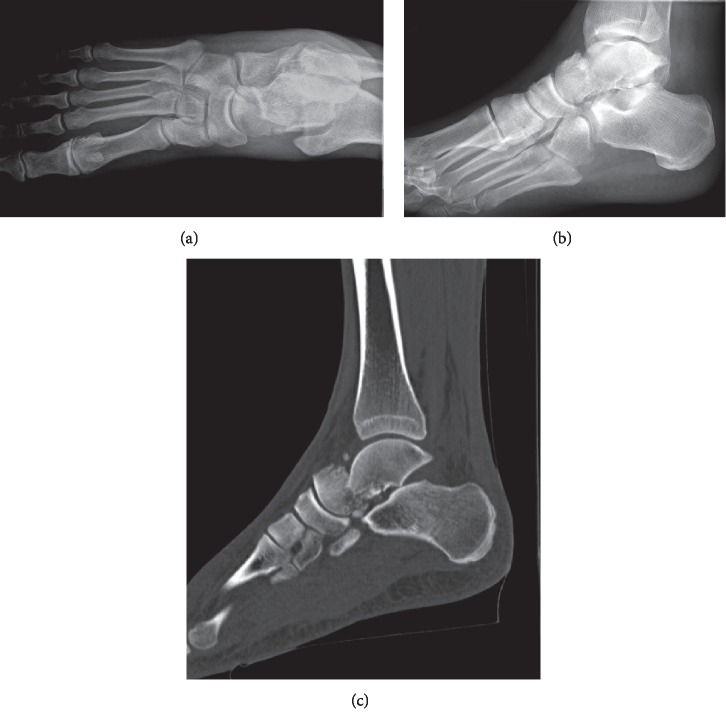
AP (a) and lateral (b) foot X-rays demonstrating a displaced talar neck fracture. CT scan (c) better elucidates the comminution and fracture pattern.
